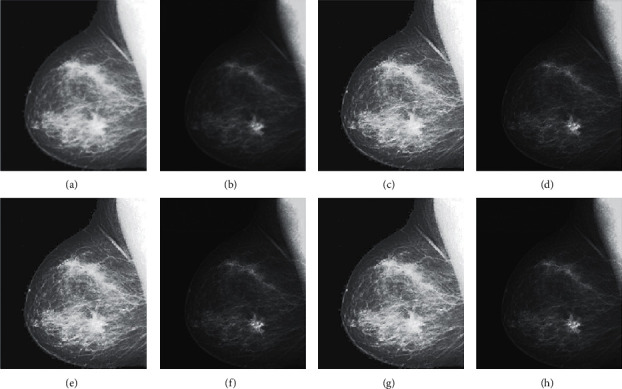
The sharpen results of “medical images” (a, b) two CT and MRI medical images, (c, d) sharpen images by Laplacian filter, (e, f) sharpened images by unsharp masking, and (g, h) LF + DF\T sharpen images.
